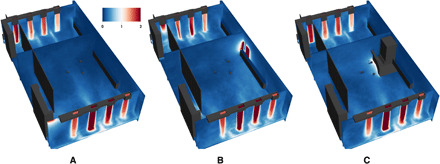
Time-averaged velocity field in Capitol Theater. Slices of the time-averaged velocity field at the level of the inlet vents, the level of the players, and mid-stage where the doors are located for scenarios CT-0 (A), CT-1 (B), and CT-2 (C). The color bar ranges from 0 m/s (dark blue) to 1 m/s (white) up to 2 m/s (dark red) on a linear scale.
